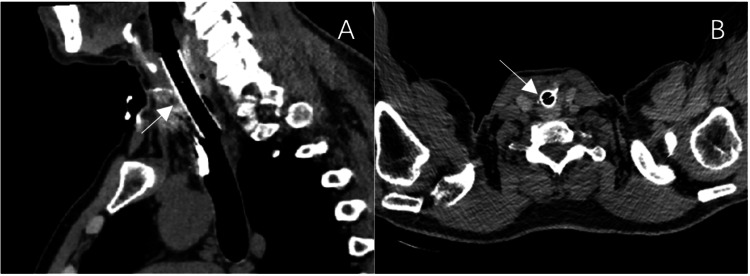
Postoperative CT imaging of the reconstructed airway (A) Three-dimensional CT reconstruction obtained on postoperative day 8 demonstrating appropriate positioning of the tracheostomy tube within the reconstructed tracheal lumen and a patent airway. (B) Three-dimensional CT reconstruction obtained on postoperative day 14 demonstrating the laryngotracheal mold in a satisfactory position without evidence of displacement.
